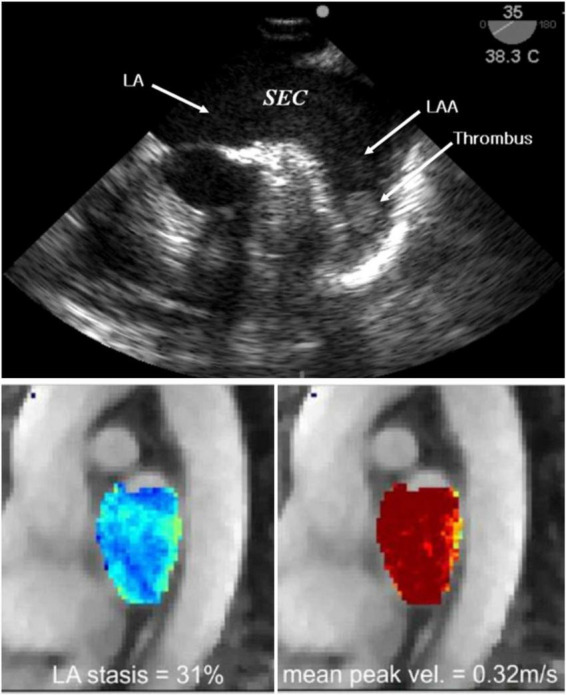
Imaging of blood stasis. 2D TEE imaging of the LA and LAA. Spontaneous echo contrast (SEC) can be seen with a dense smoke near inside the LAA indicating presence of a thrombus (top) (123). 4D Flow MRI results indicating blood stasis within the LA cavity and peak velocities at the centre and walls of the LA (bottom) (43).
